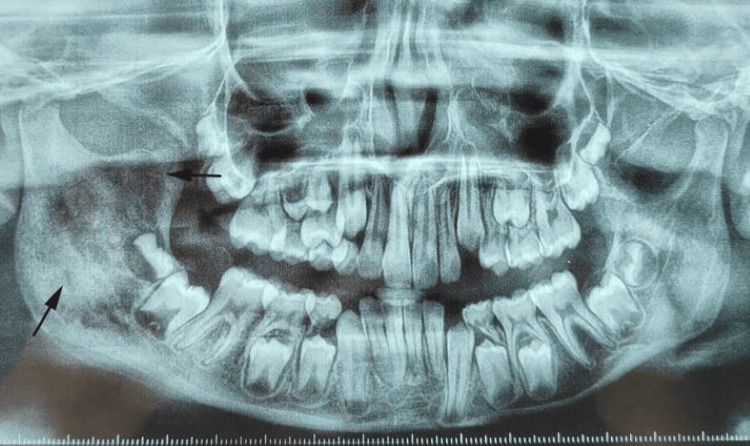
OPG showing mixed radiolucency of the ramus on the right side of the mandible OPG: orthopantomogram The current image is original and obtained by the authors
